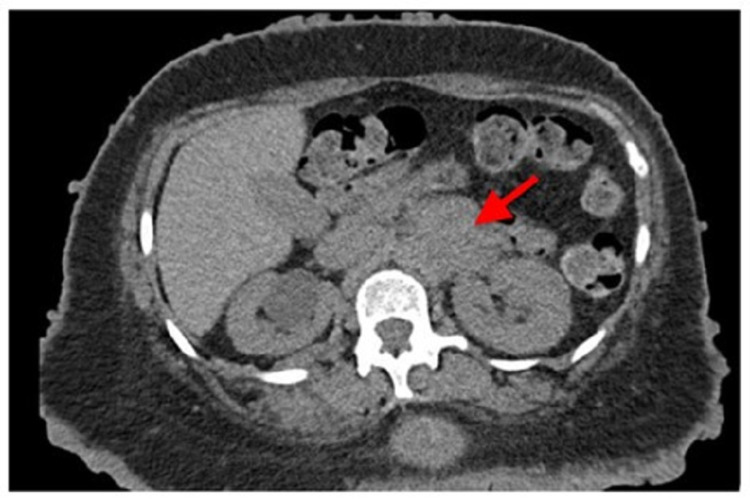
CT image demonstrating left adrenal mass (malignant peripheral nerve sheath tumor) measuring 5.5 cm × 3.9 cm.
